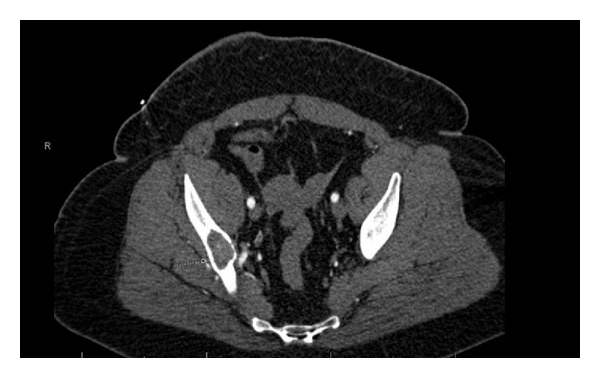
Preoperative IV contrast enhanced axial CT showing the enhancing mass in the right iliac bone.
